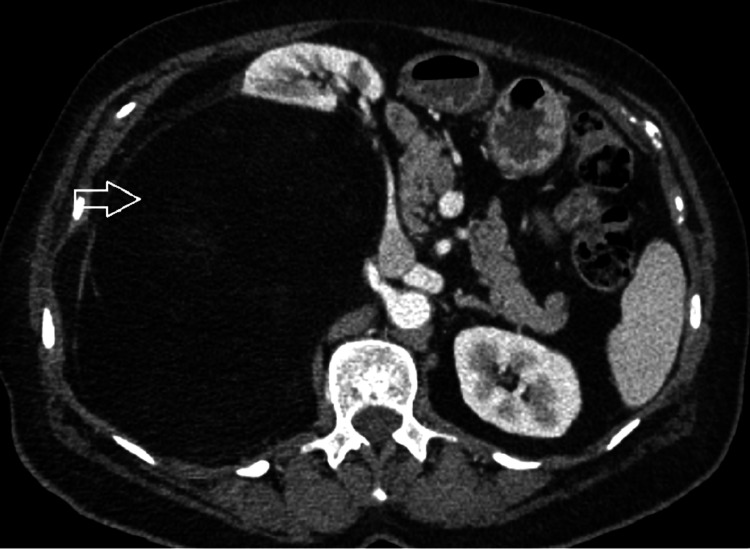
Abdominal CT scan with iv contrast showing a right retroperitoneal mass (white arrow) displacing the right kidney and the right colon anteriorly CT scan showing the retroperitoneal liposarcoma.
